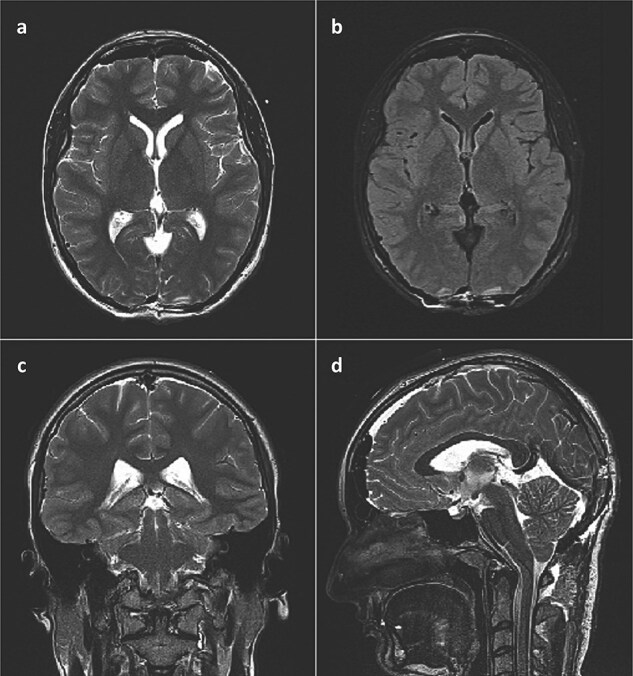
Postoperative brain MRI scan showing no residual tumor. (a) T2 weighted image, axial slice; (b) FLAIR image, axial slice; (c) T2 weighted image, coronal slice; (d) T2 weighted image (SPACE), sagittal slice).
